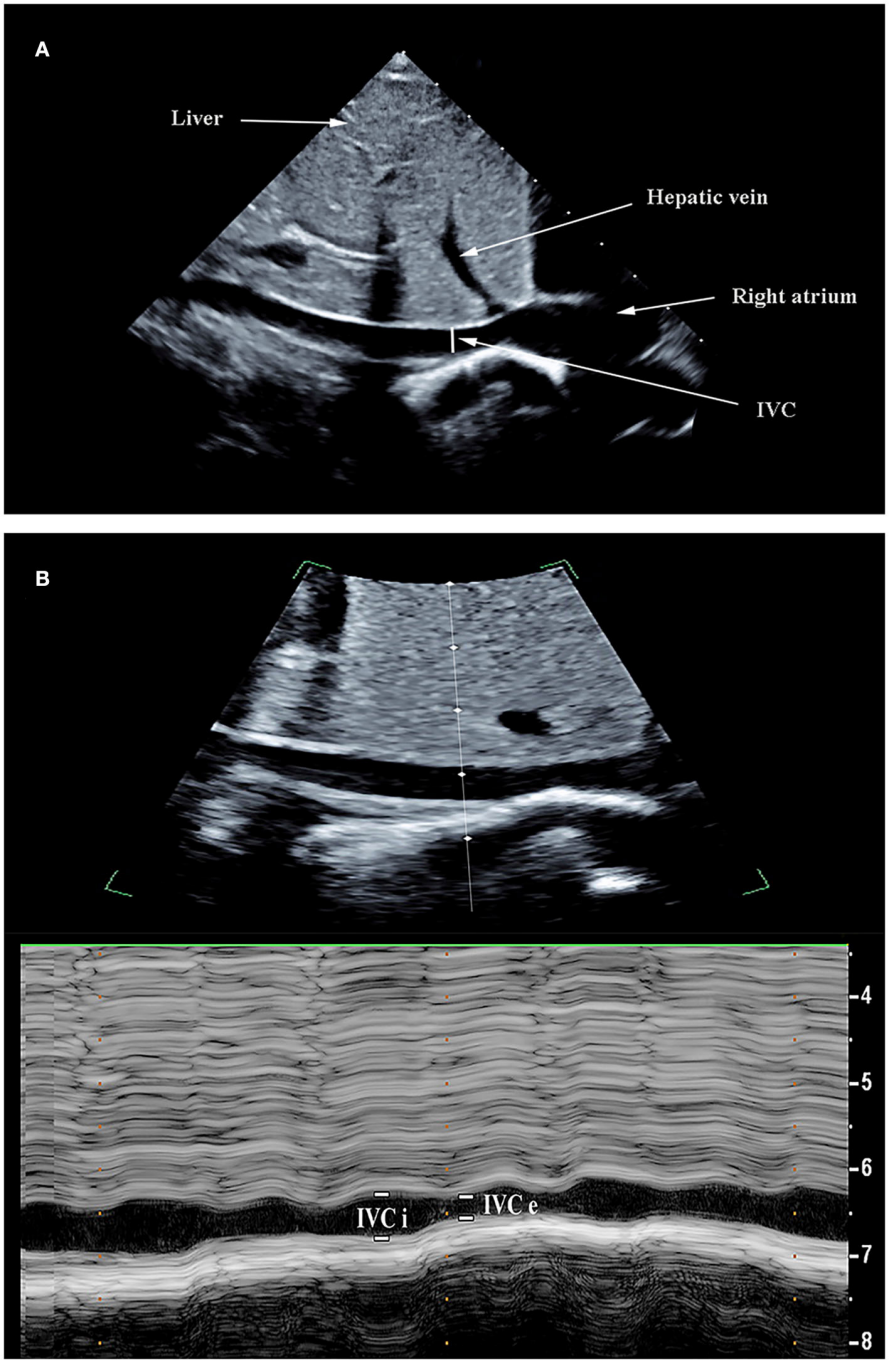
Ultrasonographic measurement of inferior vena cava (IVC) diameters. The IVC images were obtained using the bi-dimensional mode on a subcostal long-axis view (A). M-mode line was placed through the IVC 1–2 cm caudal from the hepatic vein-IVC confluence and an M-mode tracing obtained. The IVC maximum diameter (IVCmax) and IVC diameter (IVCmin) was measured during the respiratory cycle (B).
